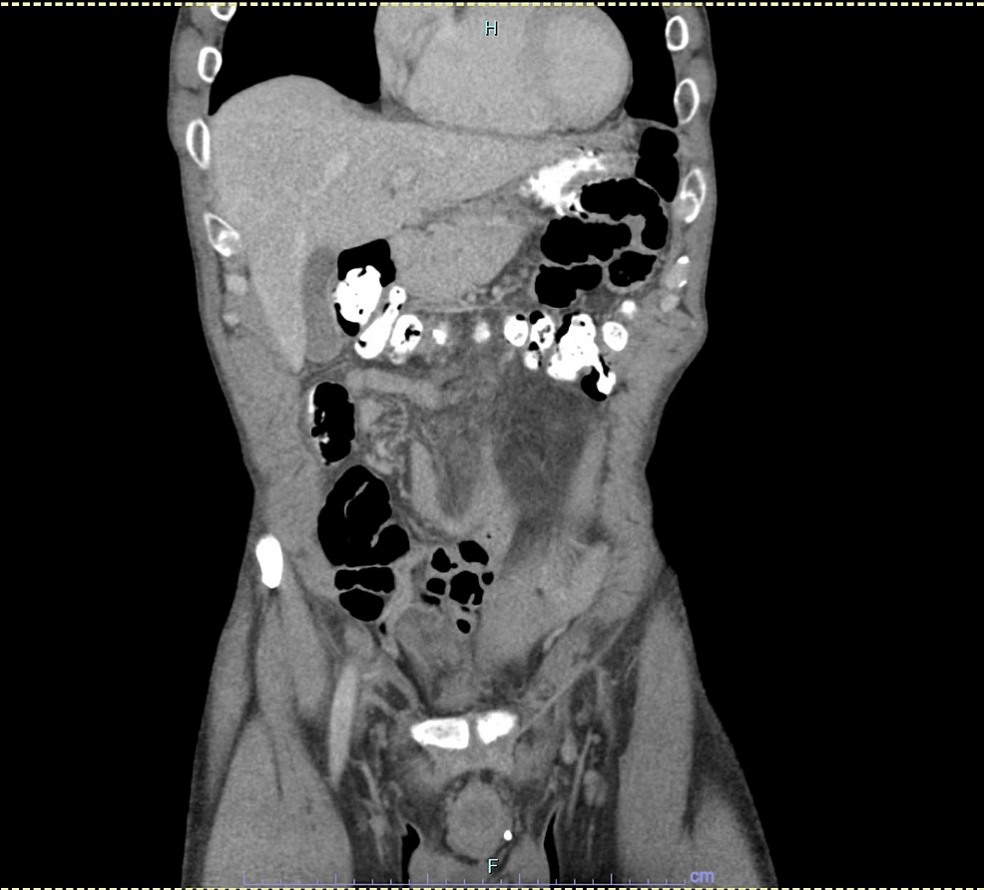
Abdominal CT scan after resection and chemotherapy showing resolution of small bowel obstruction and lymphadenopathy.
